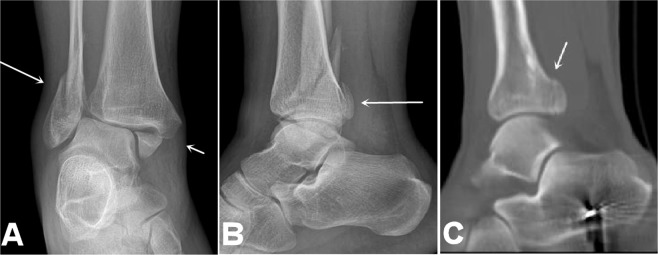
Lateral and medial malleolus fractures. (A) Anteroposterior radiograph shows lateral and medial malleolus fractures with inhomogeneous density at the distal tibial end which suggests posterior malleolus fracture. The inferior tibiofibular joint space is increased. B&C. Lateral radiograph (B) reveals the posterior malleolus fracture (B) which is confirmed by computed tomographic scan (C). This is stage 4 of the Weber type B fracture or of the supination-external rotation of the Lauge-Hansen classification.
